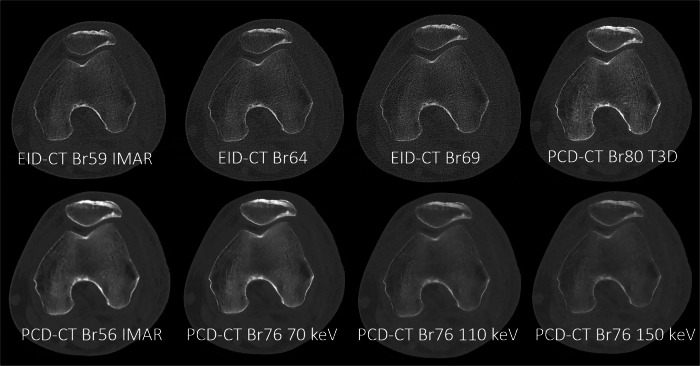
Representative axial CT images of sharp kernels centered over the distal femur
